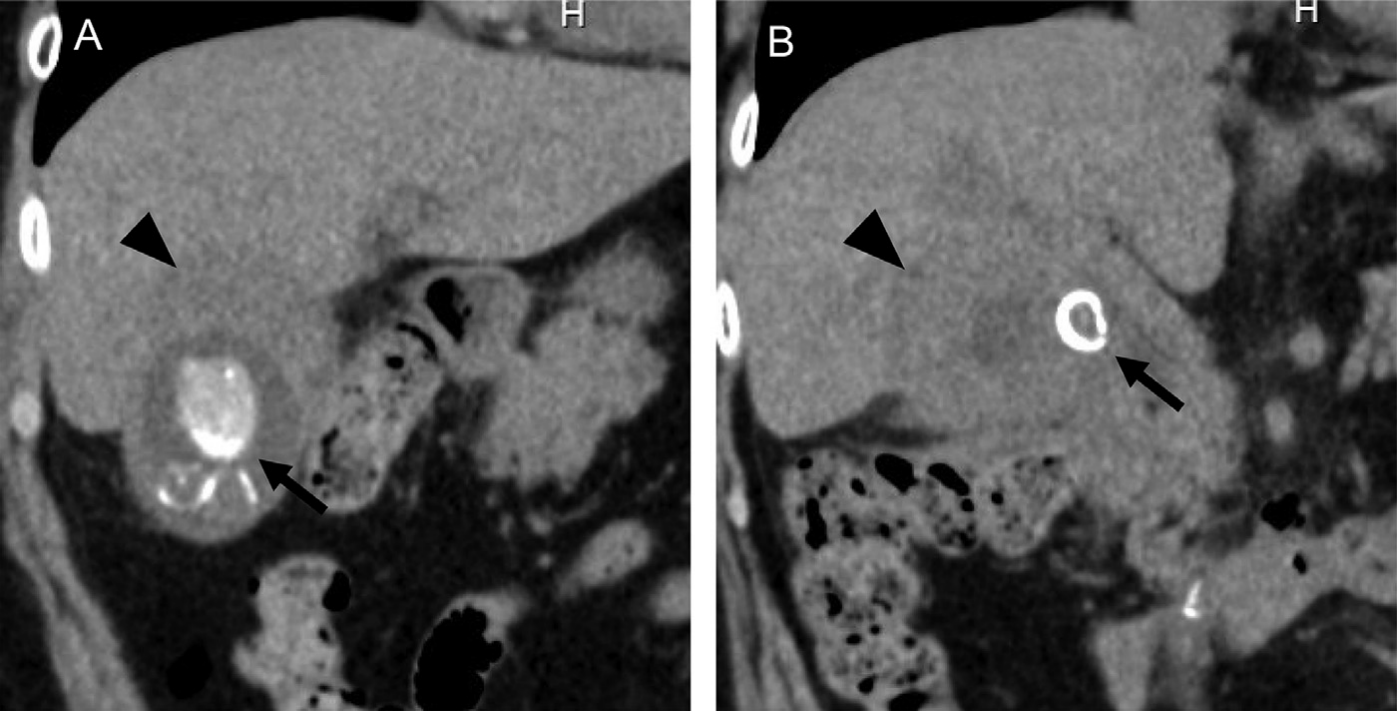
(A) Coronal image of abdominal plain CT shows multiple gallbladder stones (arrow) and wall thickening (arrowhead). (B) Another coronal image of abdominal plain CT shows a stone just right outside the bile duct (arrow). CT, computed tomography.
